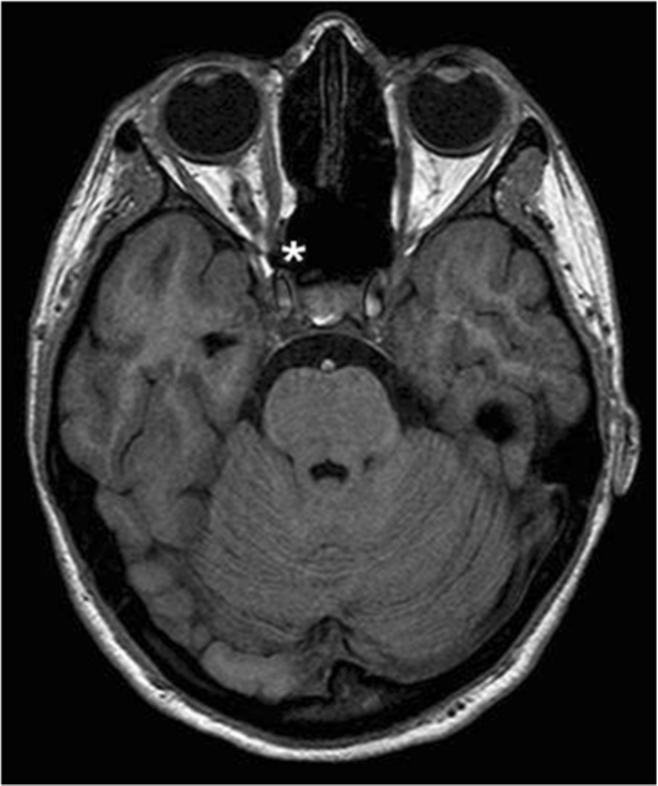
Pathology of the right optic nerve (*) – postcontrast magnetic resonance imaging.
